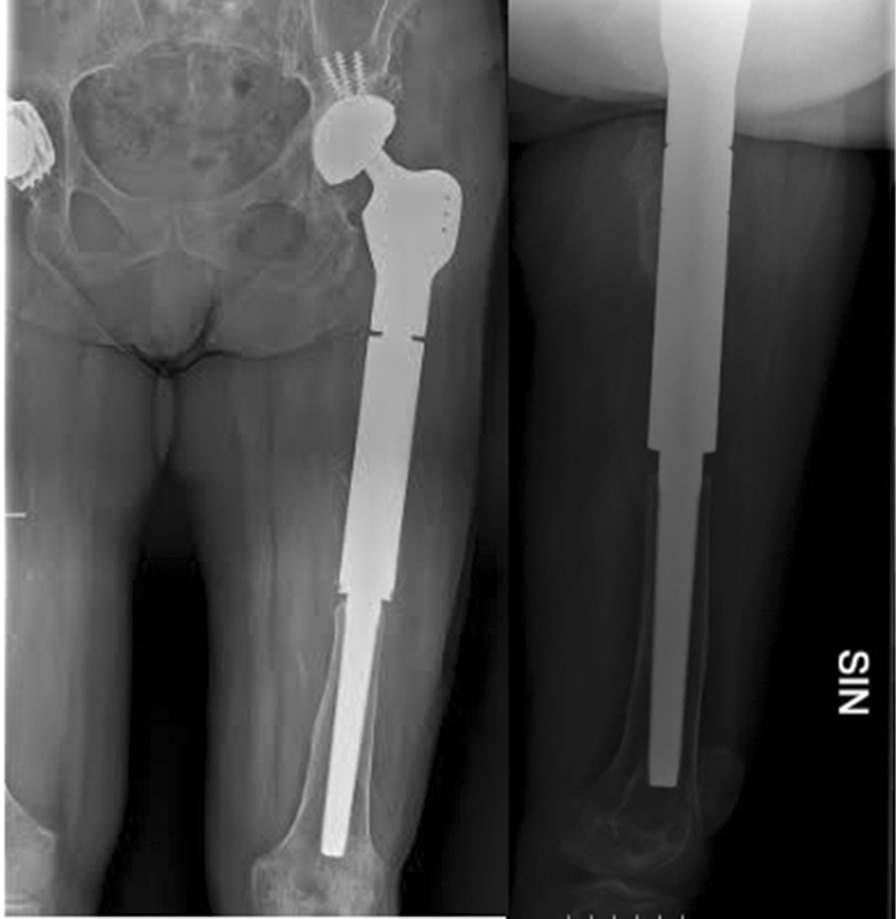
Case no. 20: Postoperative anteroposterior and lateral X-ray of a megaprosthesis
